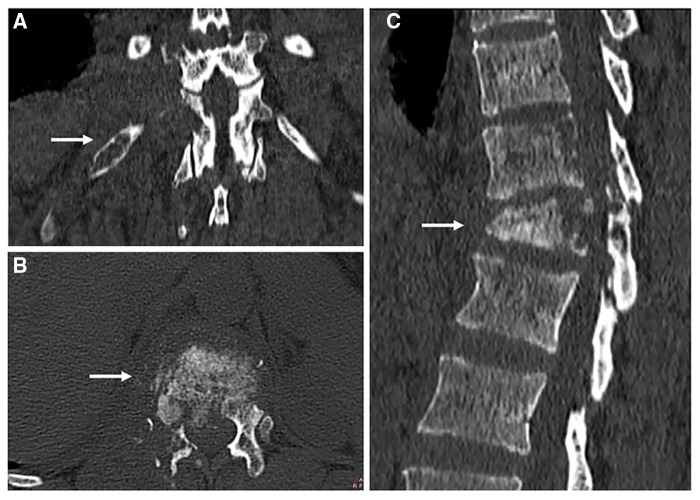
Computed tomography scans of the thoracolumbar spine. Local magnification of cross-sectional (A), coronal (B) and sagittal images. White arrows indicate destruction of vertebrae (A,C), and destruction of the 12th rib (B).
